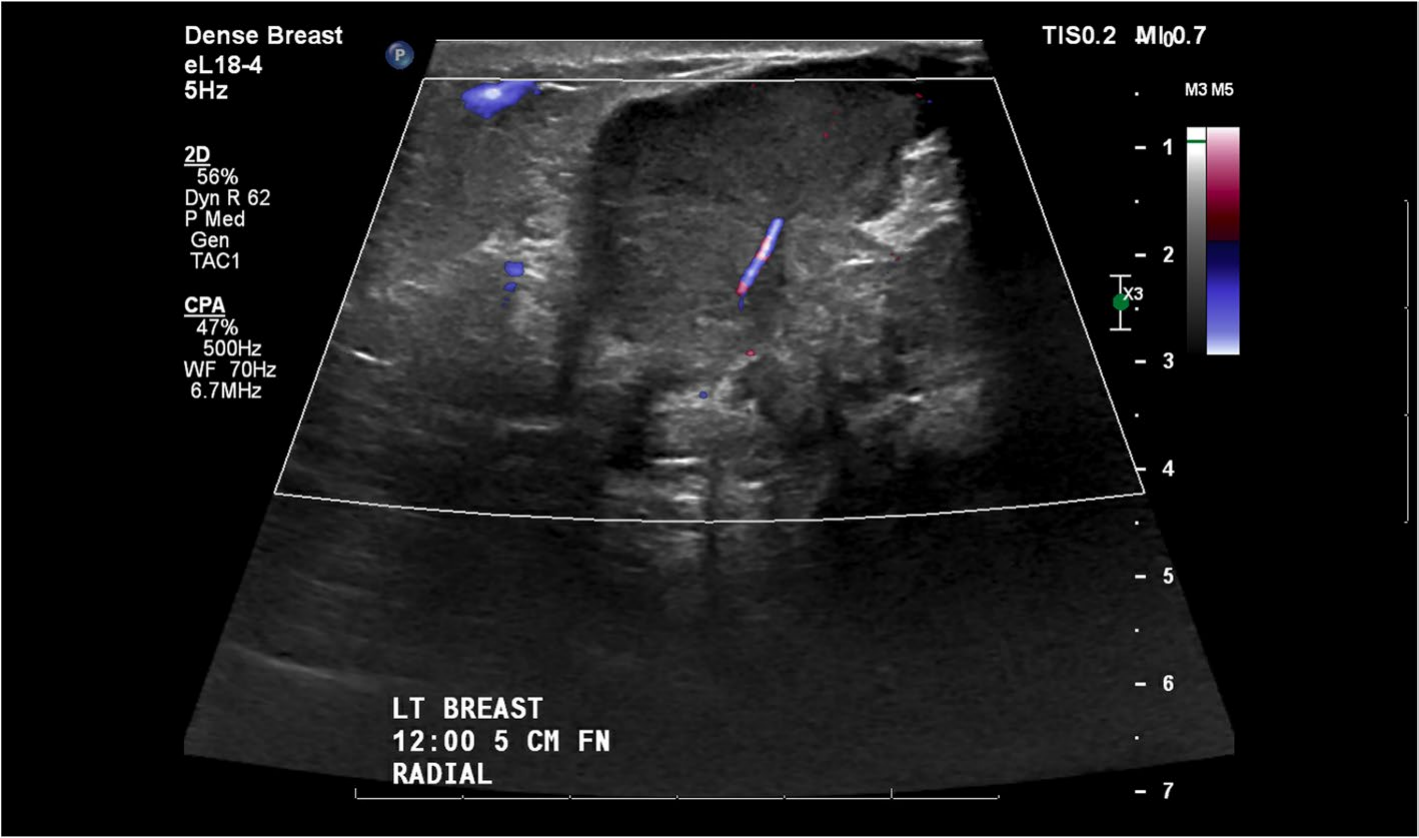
Targeted breast ultrasound demonstrates an irregular heterogenous hypoechoic mass with associated vascularity in left breast at the 12’o’clock position 5 cm from the nipple
